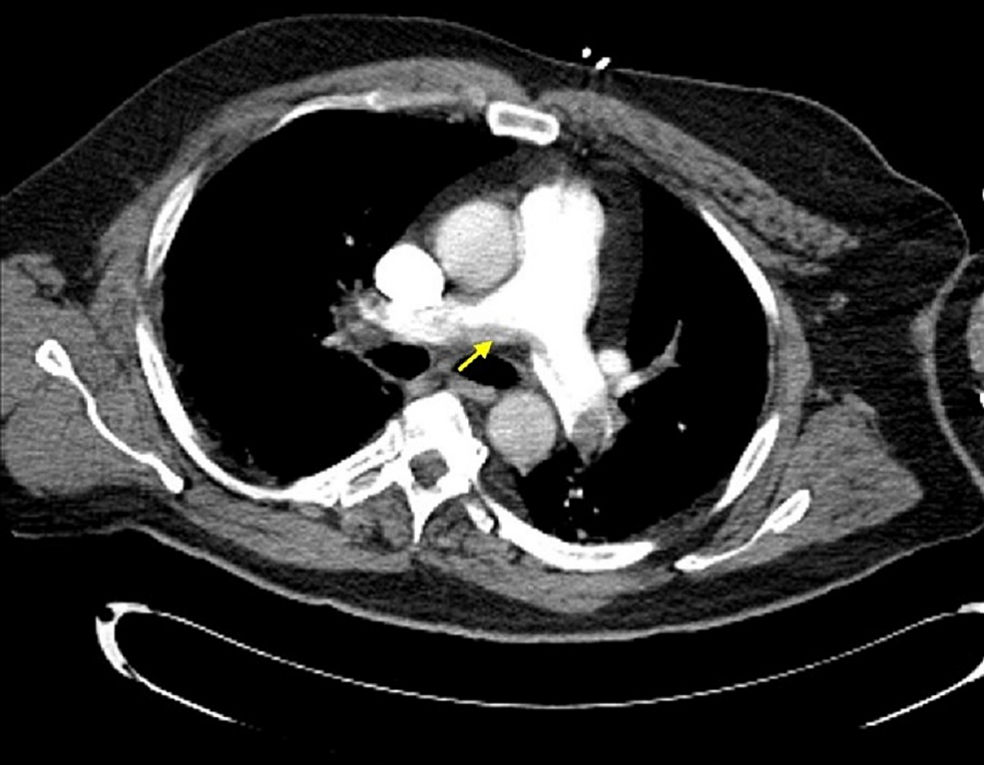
Computed tomography angiography of the chest Representative cross-sectional view. Saddle pulmonary embolus indicated by yellow arrow.
